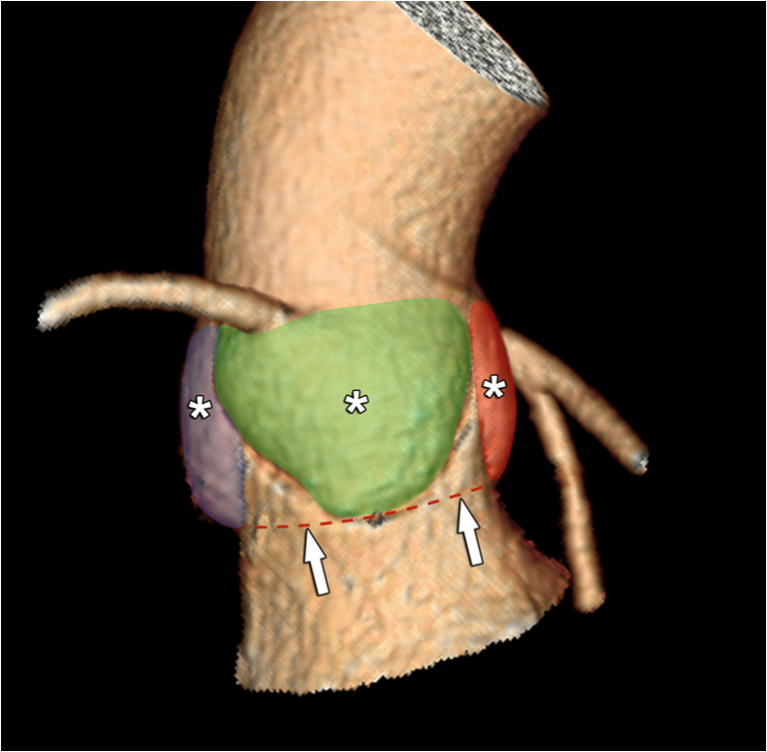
3D CT image of the aortic root containing the sinuses of Valsalva (asterisk). As the aortic valve leaflets extend within these sinuses up to the sinotubular junction, connecting their most basal attachment sites forms a virtual ring which is named the aortic annulus (red dotted line, arrows). It also marks the transition to the LVOT
